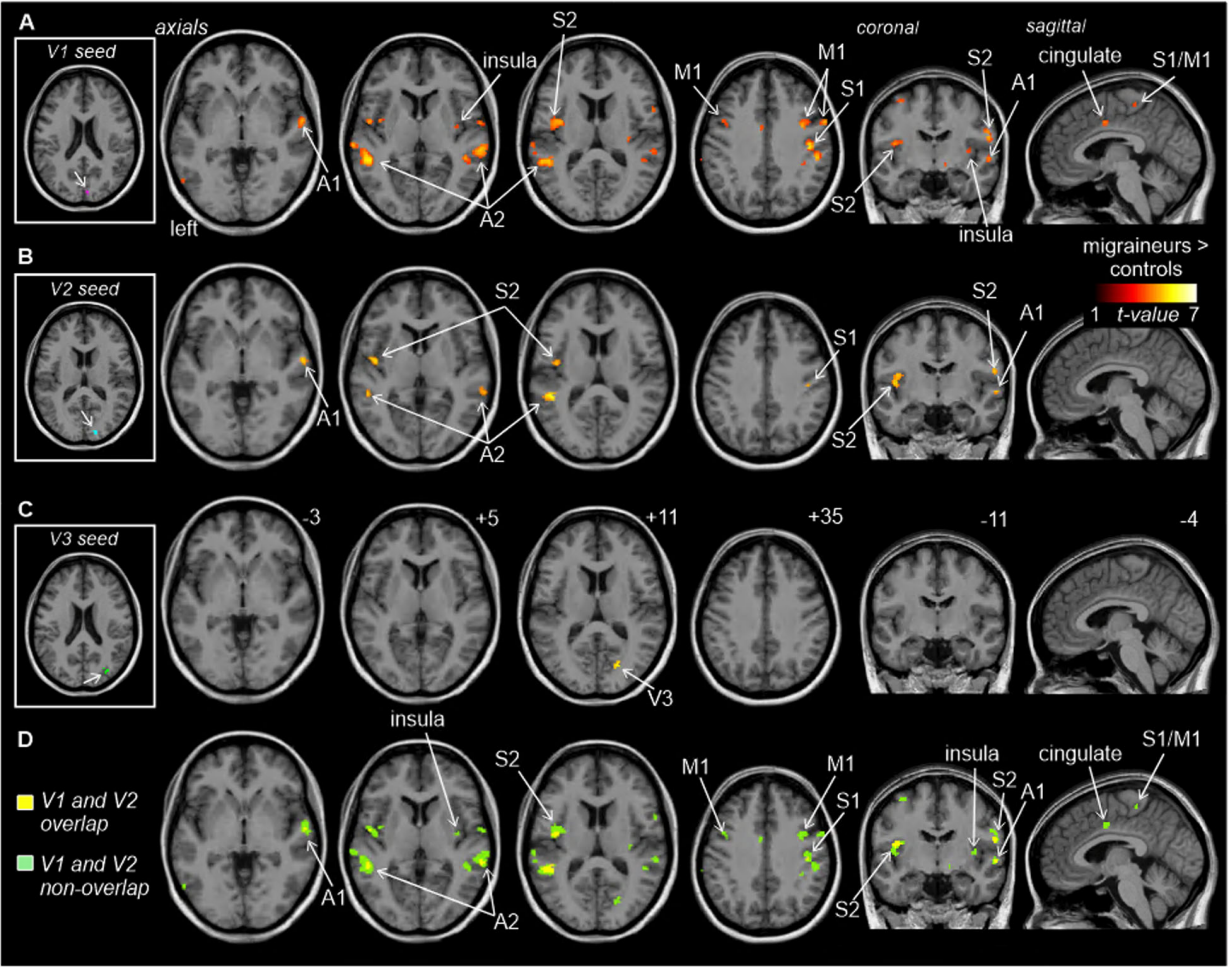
Significant differences in resting right V1, V2 and V3 connectivity between controls (*n* = 71) and migraineurs (*n* = 32) (random effects, *p* < 0.05, corrected). A. (left) An overlay of the right V1 seed extracted from the ALFF analysis (pink). Regions in which connectivity strength is significantly greater in migraineurs compared to controls overlaid on a mean T1-weighted anatomical image set. B. (left) An overlay of the right V2 seed (blue). Significant increased connectivity in migraineurs compared to controls, overlaid on a mean T1-weighted anatomical image set. C. (left) An overlay of the right V3 seed (green). Significant increased connectivity in migraineurs compared to controls, overlaid on a mean T1-weighted anatomical image set. D. Overlap (yellow) and non-overlap (green) of significant clusters in V1 and V2 functional connectivity analyses, overlaid on a mean T1-weighted anatomical image set. Location of each axial, coronal and sagittal slice in Montreal Neurological Institute (MNI) space is indicated at the top right of each slice. Slice locations are the same for all three seeds. *A1: primary auditory cortex; A2: secondary auditory cortex; M1: primary motor cortex; S1: primary somatosensory cortex; S2: secondary somatosensory cortex; V1: primary visual cortex; V2: secondary visual cortex; V3: third visual complex*
